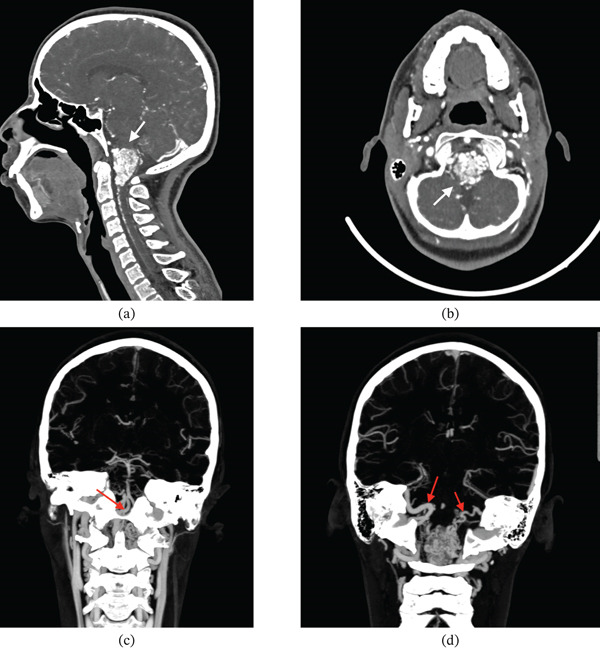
CT angiography demonstrating an arteriovenous malformation nidus at the cervicomedullary junction with serpiginous enhancing vessels. (a) Sagittal reformatted image showing the nidus (white arrow). (b) Axial image confirming the nidus at the cervicomedullary junction (white arrow). (c) Arterial supply arising from the anterior spinal artery (red arrow). (d) Early venous drainage into the bilateral sigmoid sinuses (red arrows).
